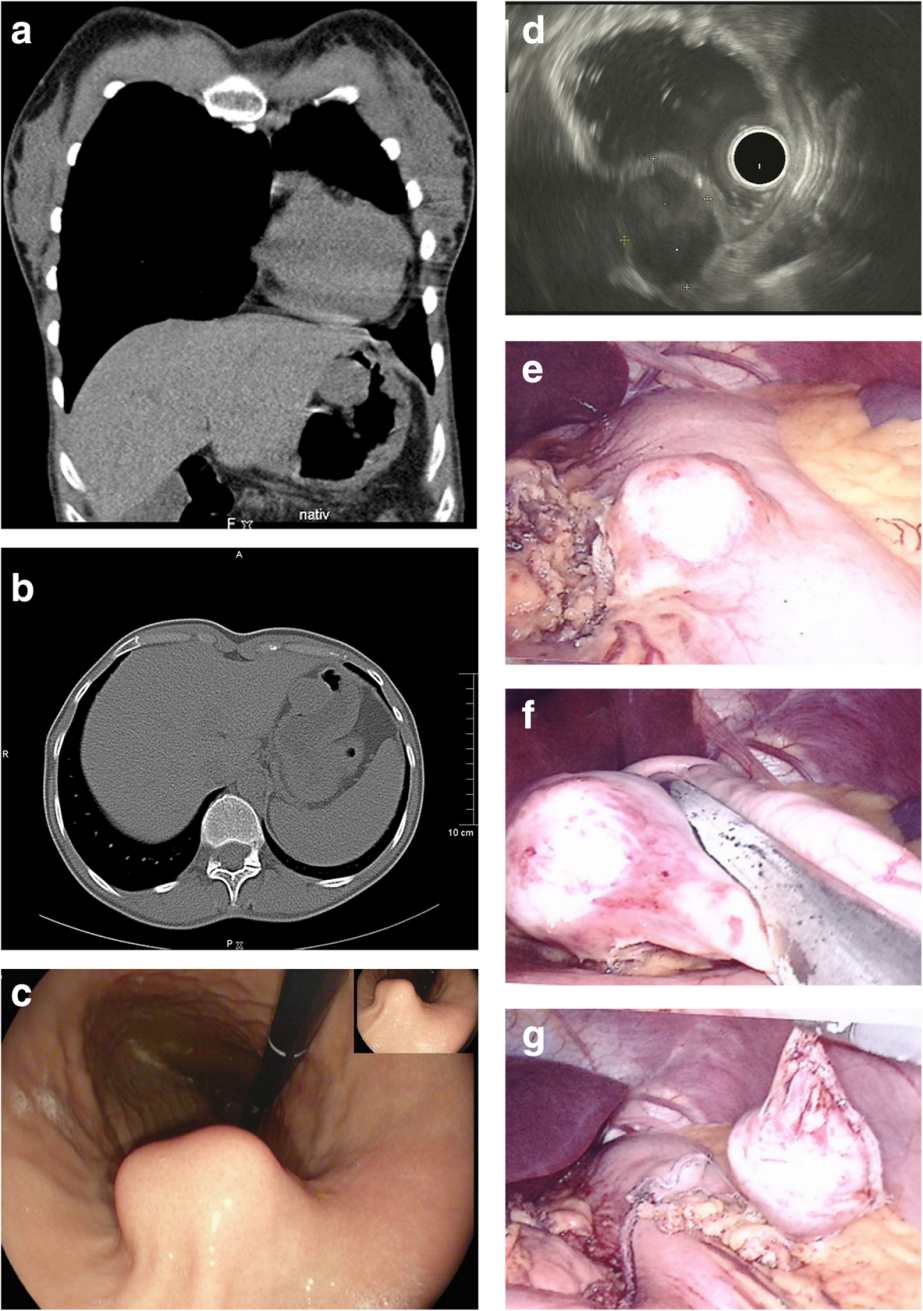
a, b. Incidental contrast-enhanced computed tomography (CT) finding shows a round, exophytic, well-defined, homogeneous mass (30 mm) with contrast enhancement, arising from the lesser curvature of the gastric body. c GI endoscopy submucosal mass (26 mm × 22 mm) with smooth overlying normal mucosa in the lesser curvature of the gastric body. d EUS picture showing a hypoechoic lesion located within the muscularis propria. e Laparoscopic intraoperative picture of an exophytic mass along the lesser curvature of the body of the stomach. f, g Laparoscopic tumor wedge resection using mechanical laparoscopic sutures
